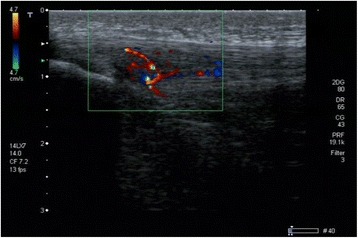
Sonographical findings in a player of the U-17 team with acute PTS in the right knee. Structural changes like hypo-echogenic areas; neovascularization and tendon thickening were all detectable
